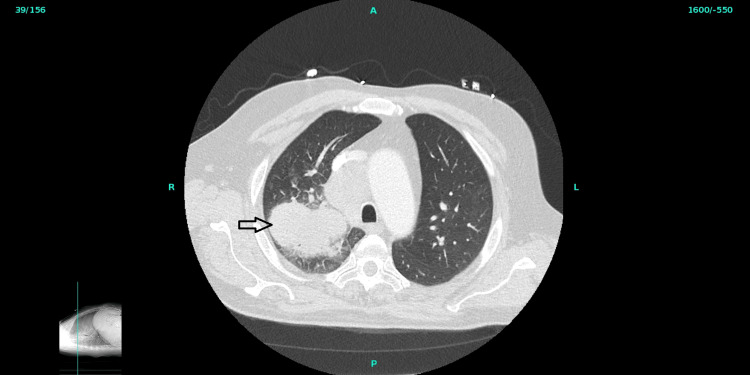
CT chest showing large right upper lobe lung mass
